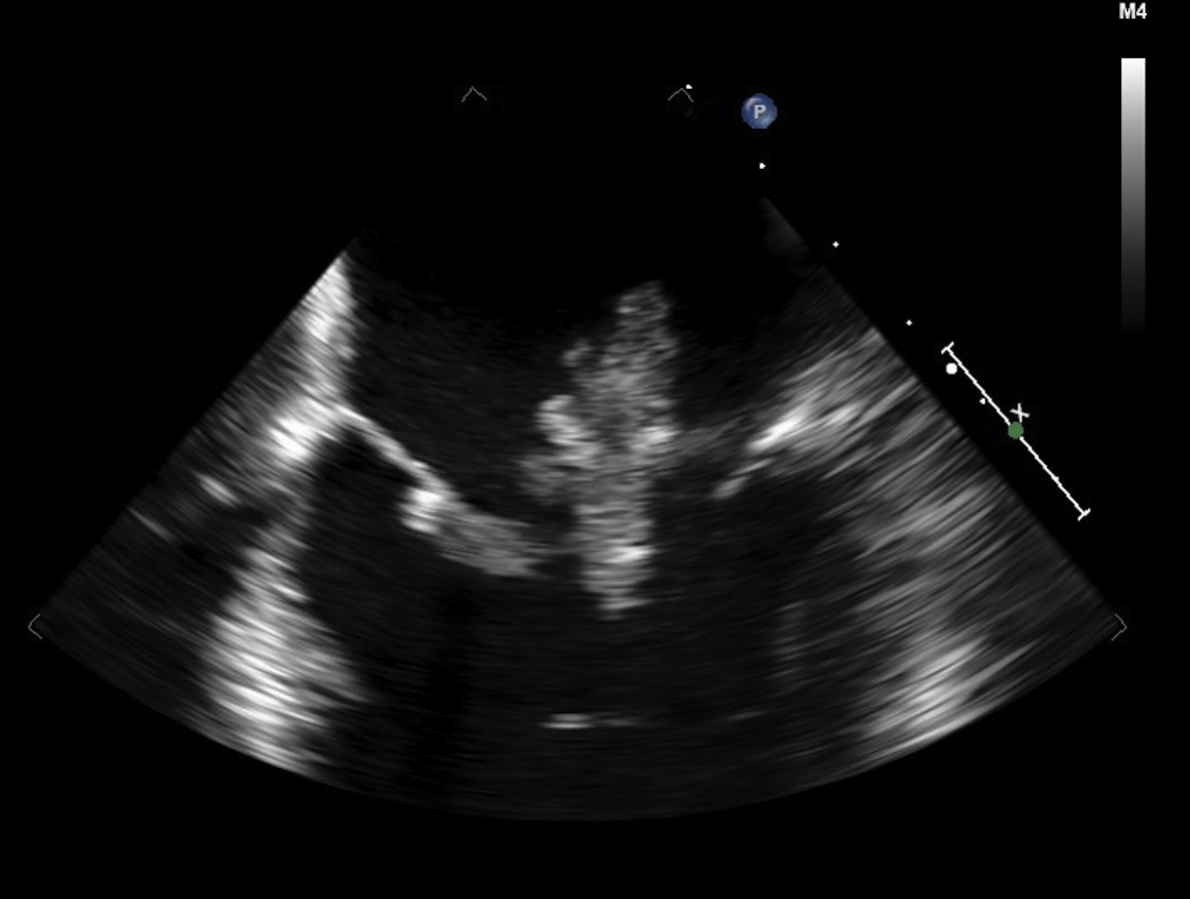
2D transesophageal echocardiogram shows a large left atrial mass that protrudes through the mitral valve during diastole.
